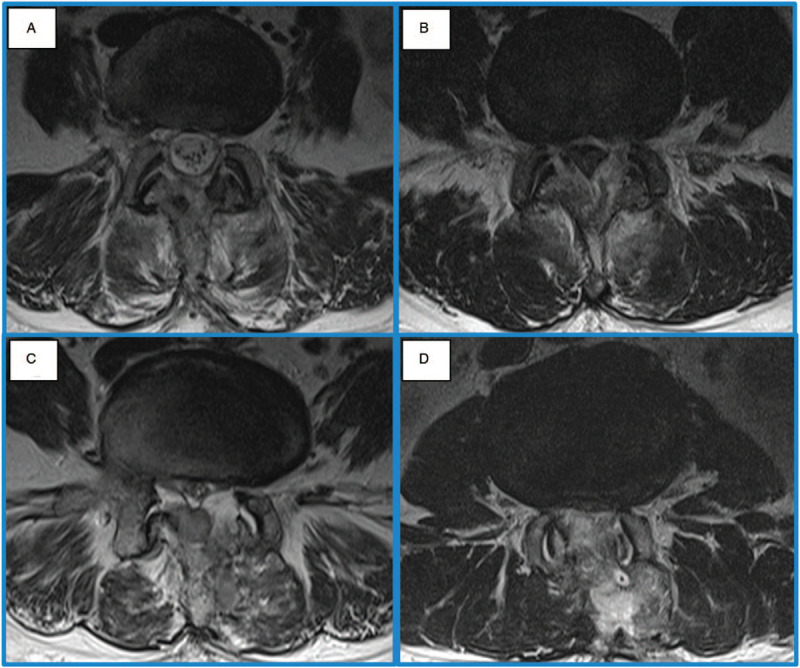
The grading system of thecal sac compression by epidural hematoma in T2 MRI axial images. (A) Grade 1; that stands for the thecal sac compression less than a quarter. (B) Grade 2; between a quarter and a half. (C) Grade 3; between a half and three quarters. (D) Grade 4; over three quarters.
